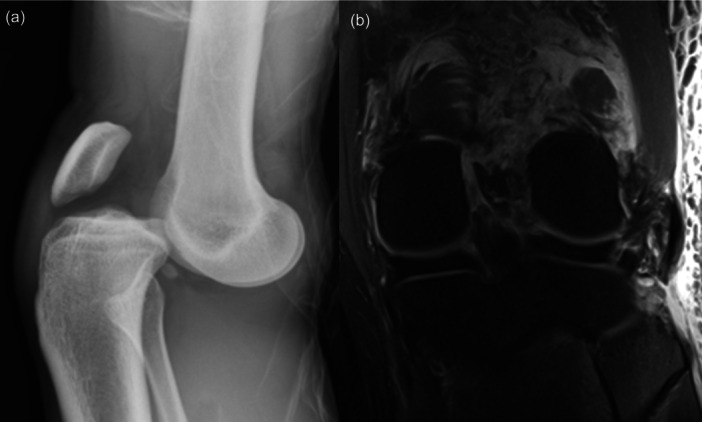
(a) X‐ray revealing an anterior knee dislocation. (B) Coronal T2‐weighted MRI image showing a KD‐III‐L with avulsion of the biceps femoris and lateral collateral ligament from the fibular head. KD, Knee dislocation; MRI, magnetic resonance imaging.
